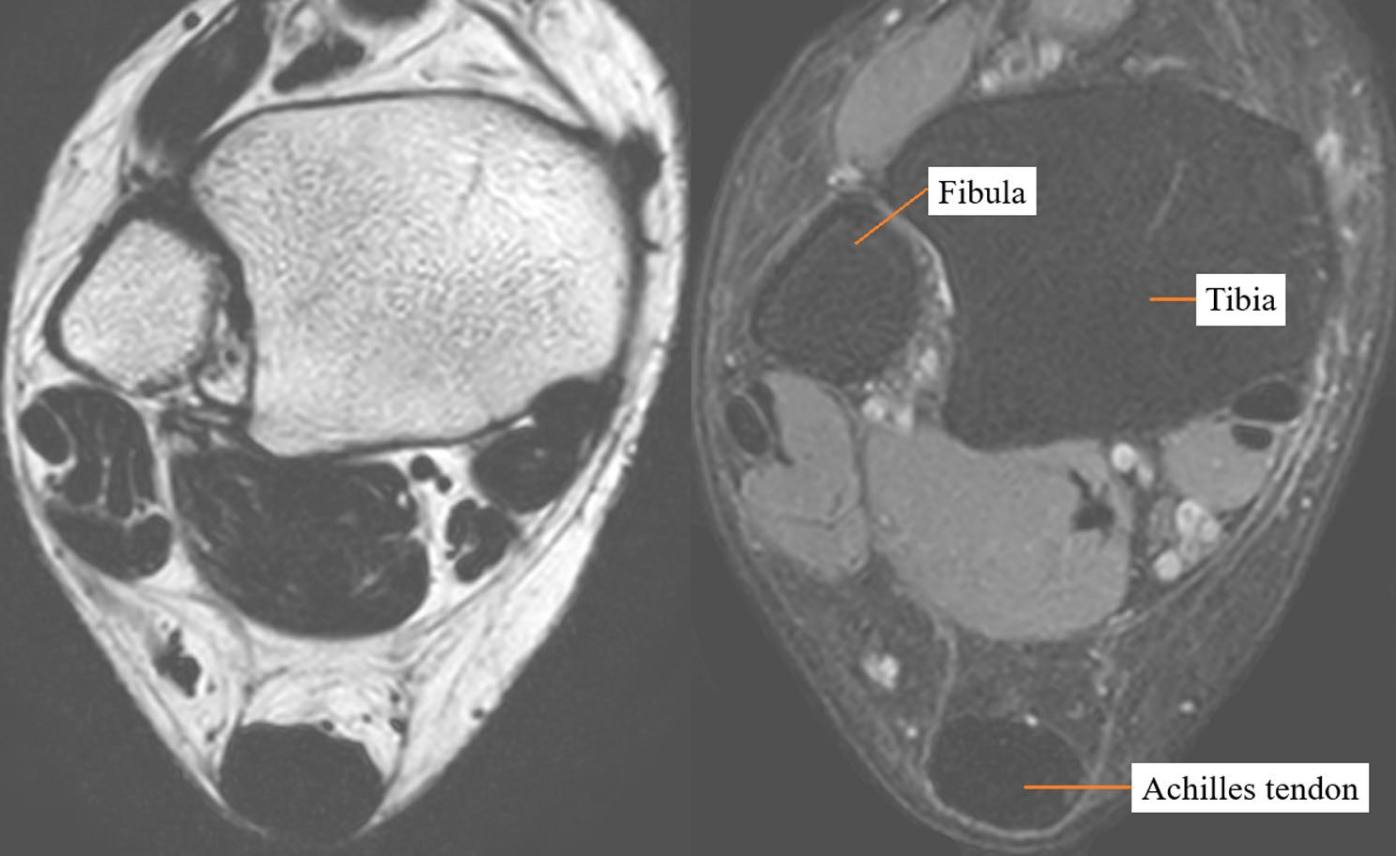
Dixon method MR imaging. Lipid-only (left), and water-only (right) sequences
